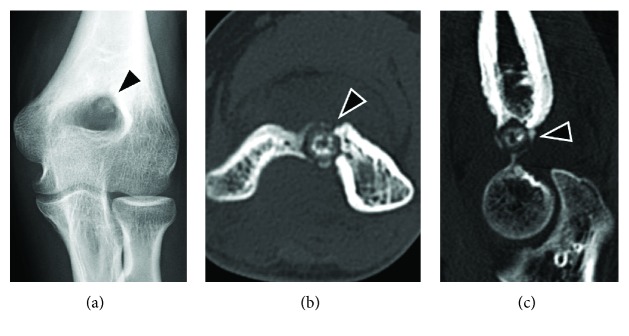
Preoperative radiographic images. The anteroposterior radiograph (a) and computed tomography images (b: axial, c: sagittal) depict a nidus comprising central sclerosis with circumferential radiolucency (arrowhead) at the olecranon/coronoid fossa of the humerus.
